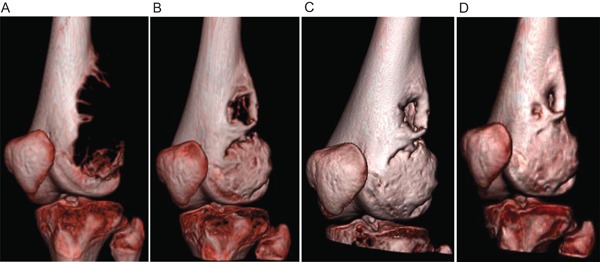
Computed tomography showing regression of the giant cell tumor of bone in patient 1. (*A*) before denosumab initiation, (*B*) 1.5 months after denosumab initiation, (*C*) 3 months after denosumab initiation, and (*D*) 8 months after denosumab discontinuation.
